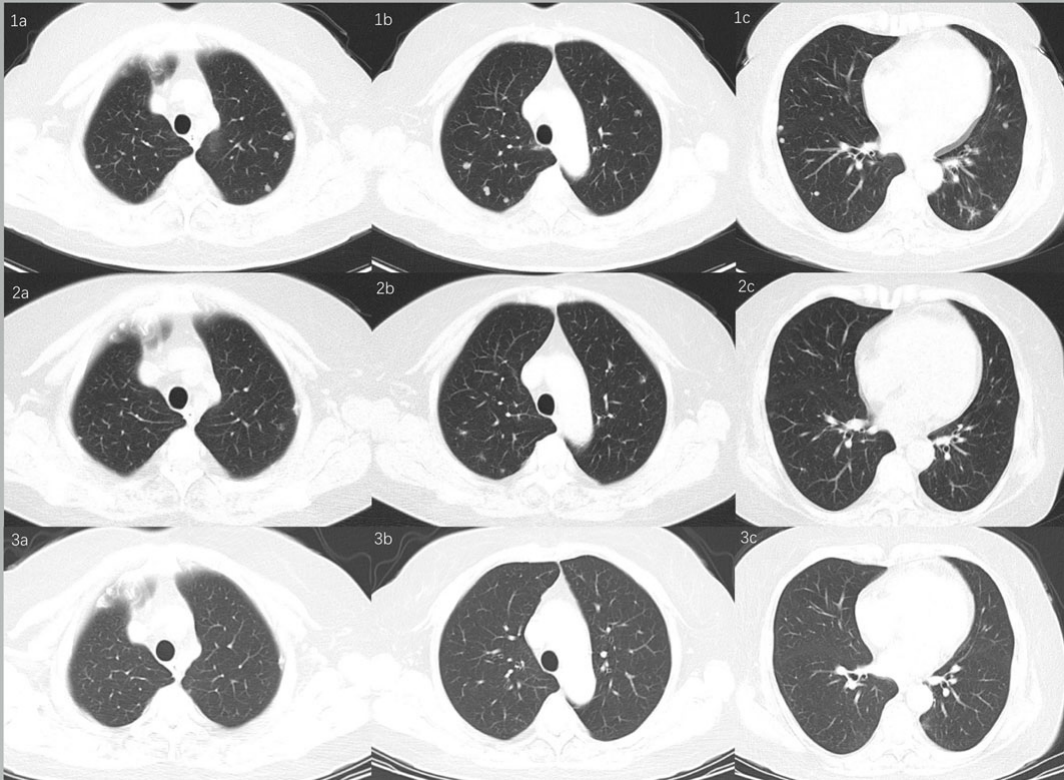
Chest CT scan showed lung metastasis. (1a–1c)
